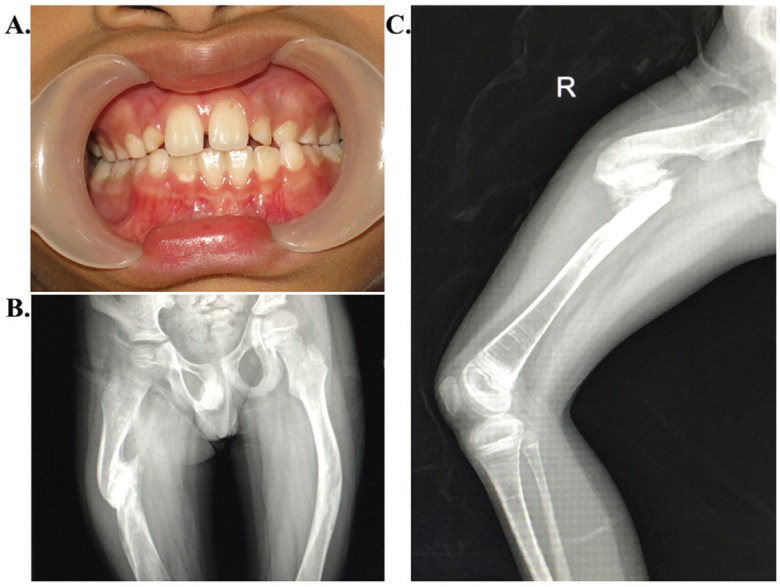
Clinical and radiographic features of the patient with osteogenesis
